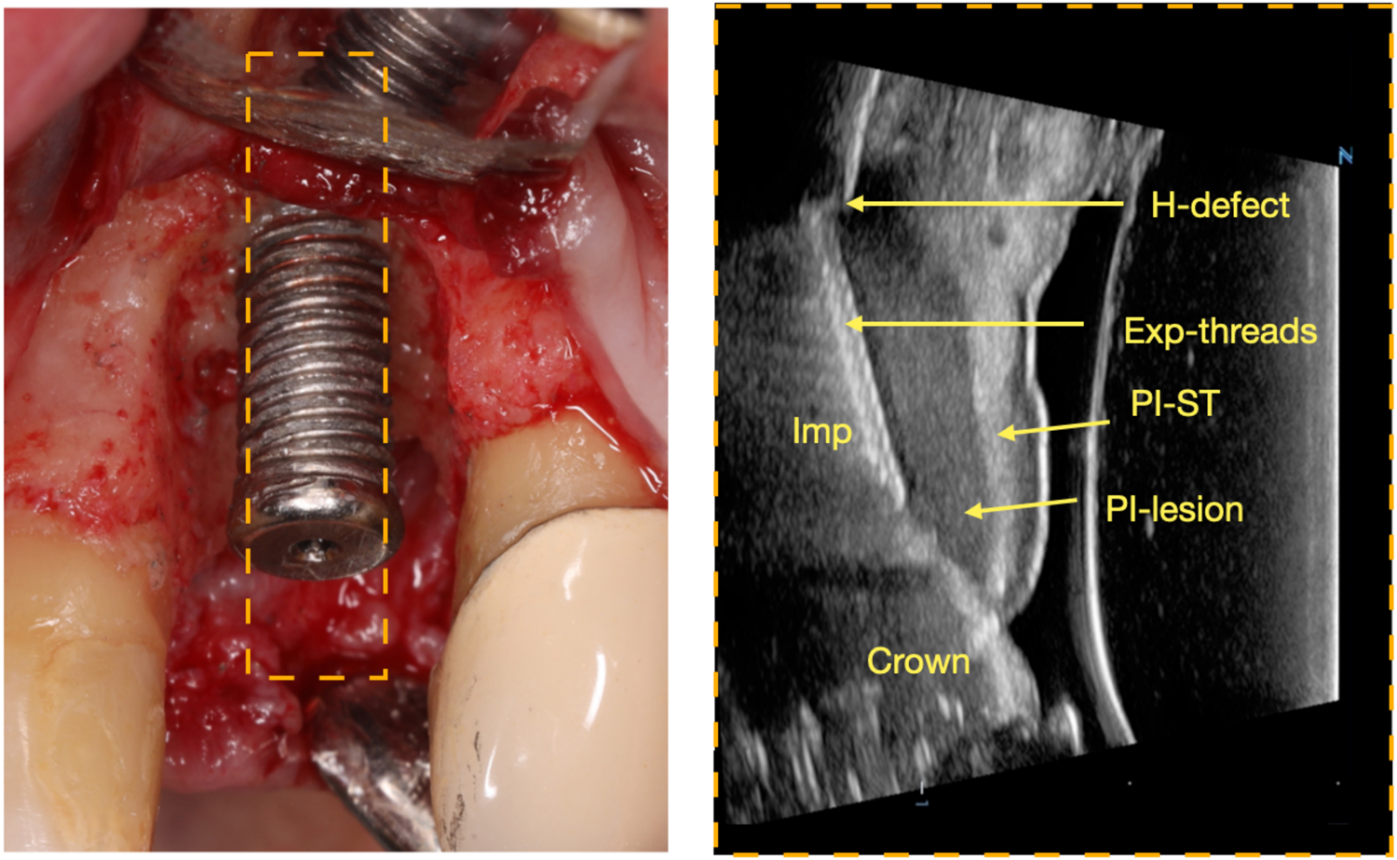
Preoperative ultrasound image (Right) before crown removal that can be used for treatment planning regarding the defect type, amount of facial bone loss, and soft tissue quality. In this case, a relative horizonal facial defect (H‐defect) was found, with exposed implant threads (Exp‐threads), peri‐implant lesion (PI‐lesion) and thick peri‐implant tissue (PI‐ST). The image was confirmed during open surgery (Left).
